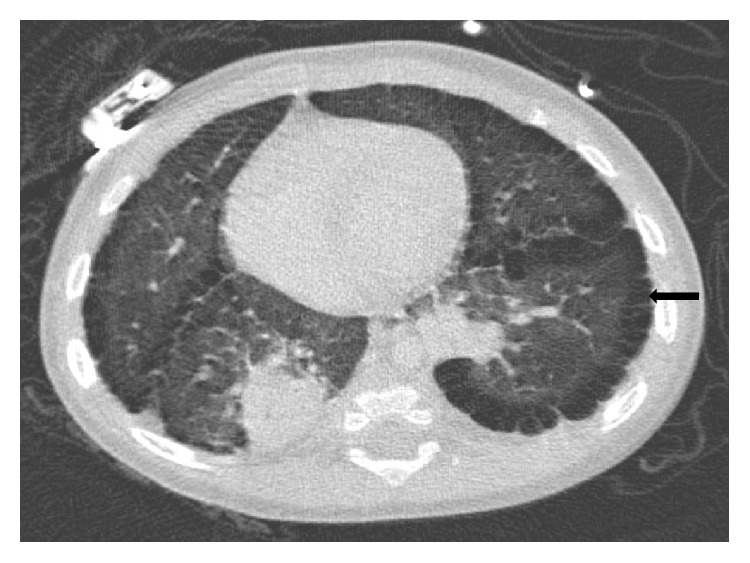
Axial view of chest CT shows the subpleural cysts (block arrow) of both lungs along the chest wall and the major fissure.
